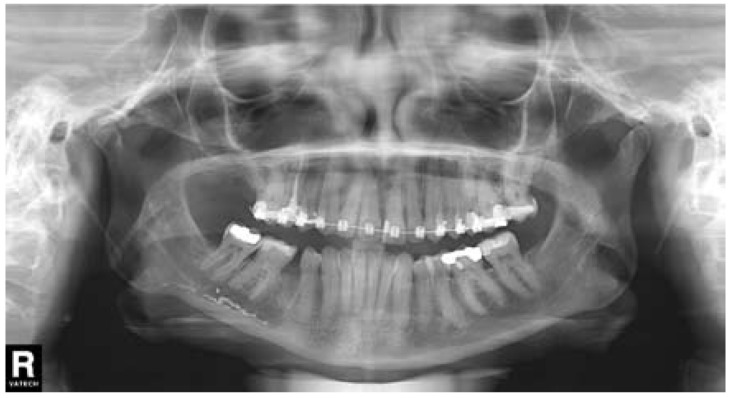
Panoramic radiograph after three weeks of treatment with pregabalin and prednisone.
